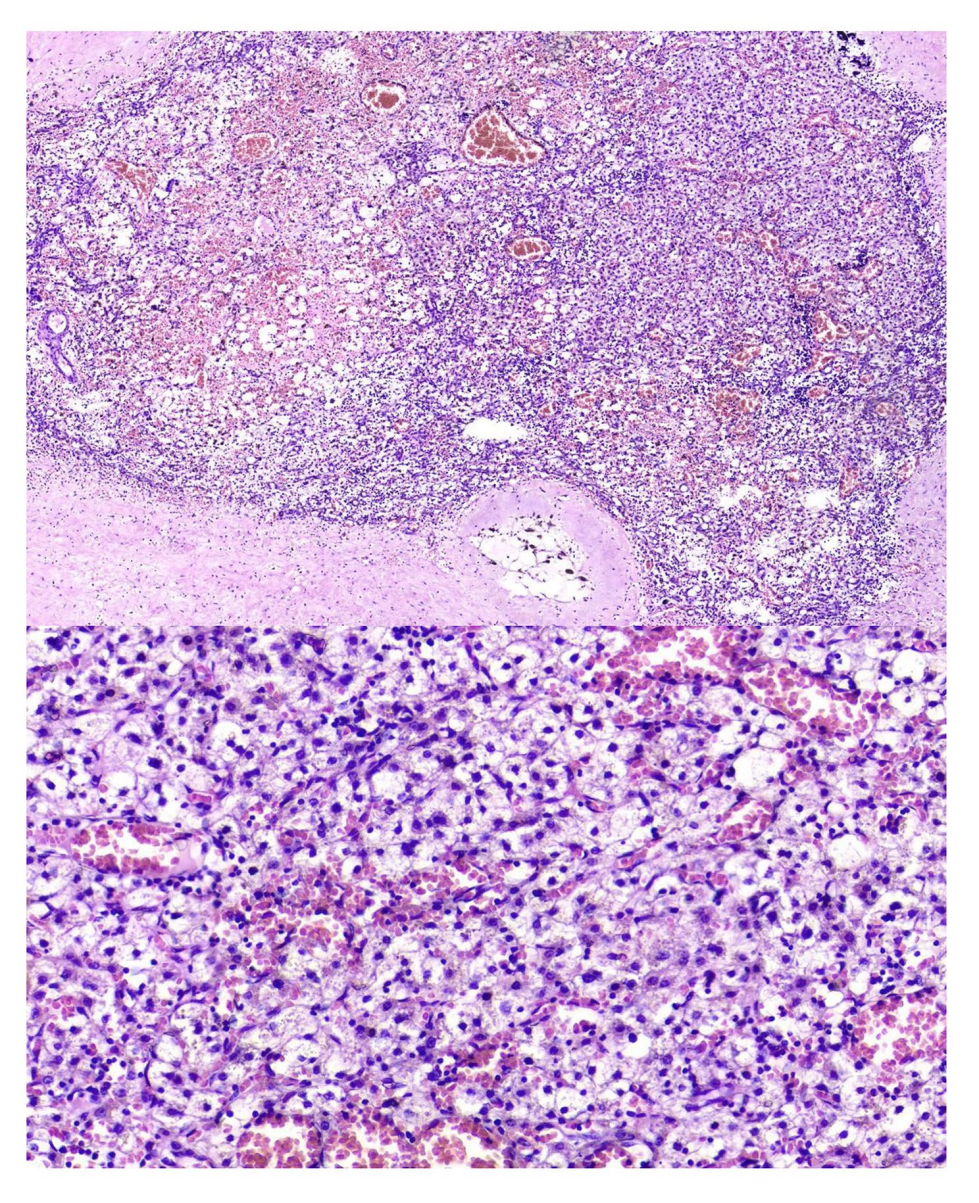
CT imaging after preoperative chemotherapy showing a significant reduction in the size of the tumor.
